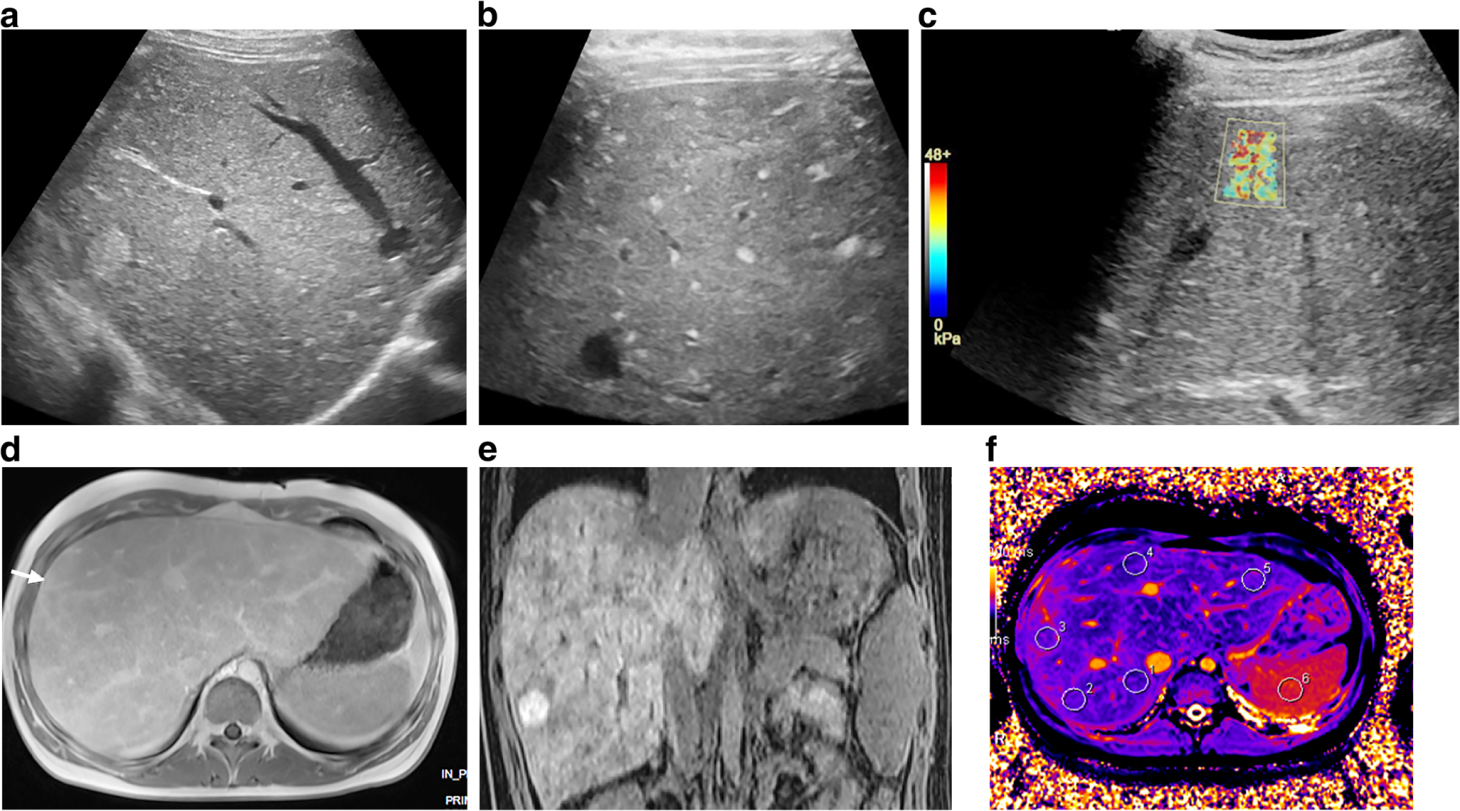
Ultrasound elastography and MRI in Fontan-associated liver disease in a 16-year-old girl with fibrosis, cirrhosis and nodular liver lesions. a Sagittal US imaging reveals a heterogeneous parenchyma and a large nodular lesion of 2 cm in diameter laterally in the right liver lobe. b, c Transverse linear high-frequency US probe reveals multiple small hyperechoic lesions (b), and transverse shear-wave elastography shows increased liver stiffness (c). d Axial T1-W gradient echo MR image post gadolinium contrast injection reveals an enlarged congestive liver with irregular enhancement of the nodule (*arrow*). e Coronal T1 gradient echo image shows strong enhancement of the nodule with gadoxetic acid in late hepatobiliary phase, interpreted as a focal-nodular-hyperplasia-type lesion. f MR native T1 mapping in an axial midsection plane, with regions of interest drawn in the liver and spleen, shows irregular increased T1 times in the periphery
